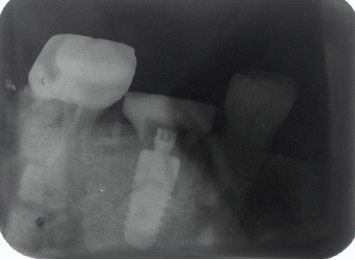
Intraoral periapical radiograph at the 4-month follow-up.
